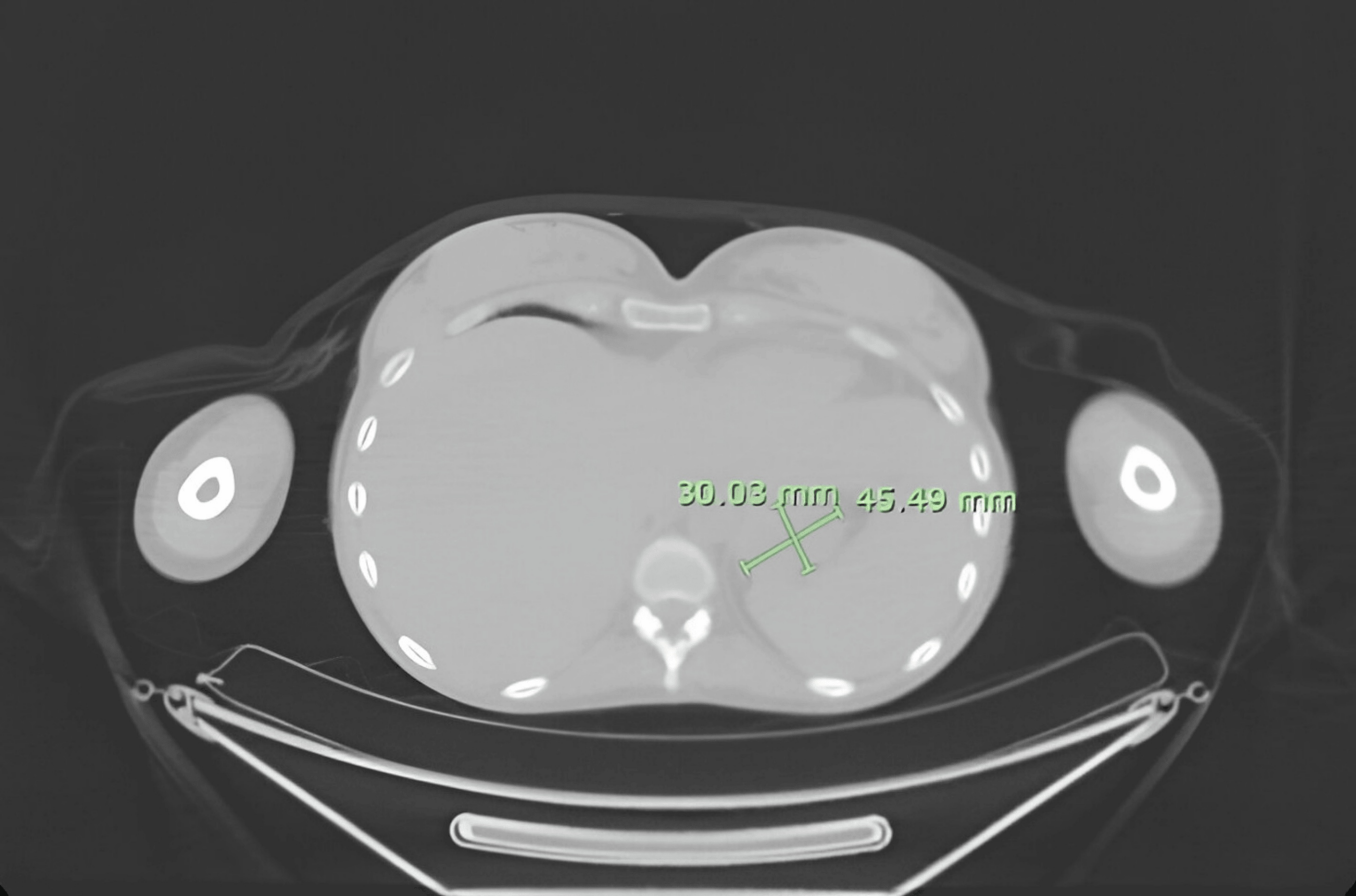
Computed tomography scan showing a gastric mass measuring 30.03 x 45.49 mm
